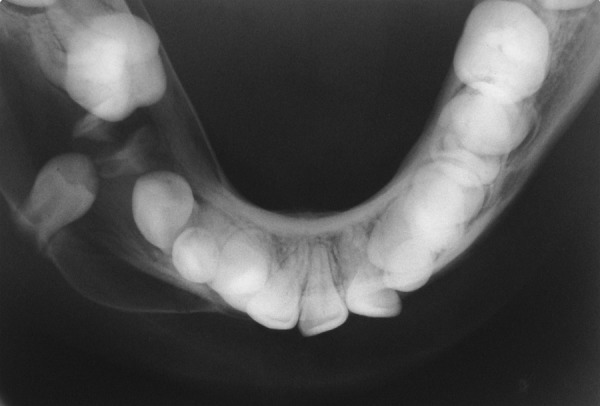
Occlusal radiograph showing expansion of buccal and lingual cortical plate
